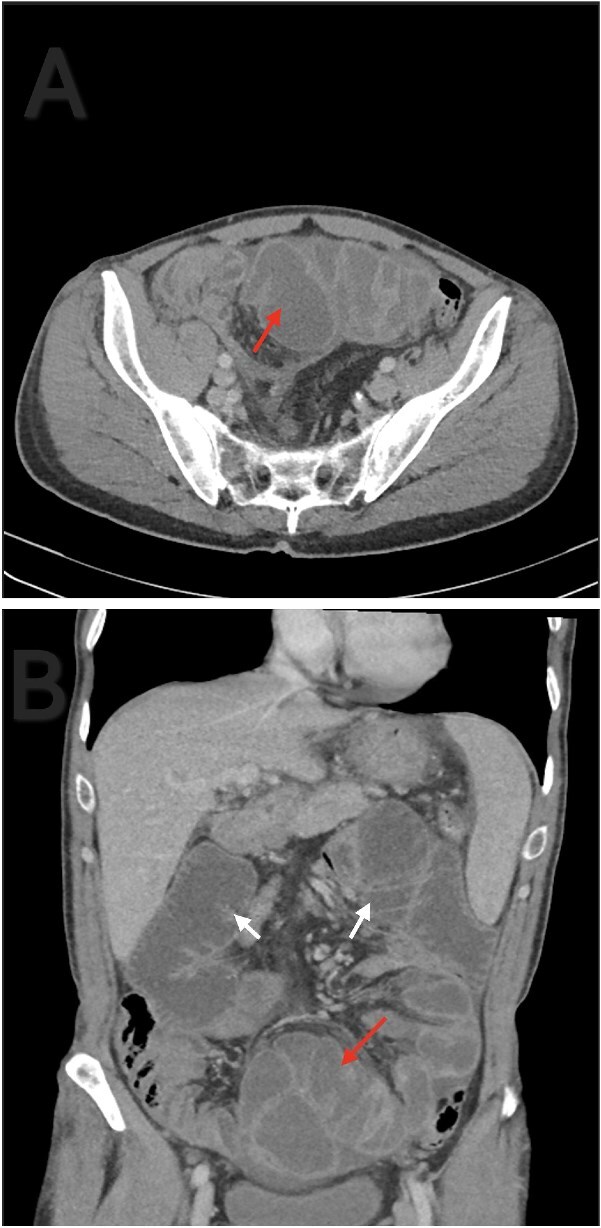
Axial (A) and coronal (B) contrast enhanced abdominal CT. Ileal loops congregated at the pelvis (red arrow). Small bowel loops dilation (white arrow).
